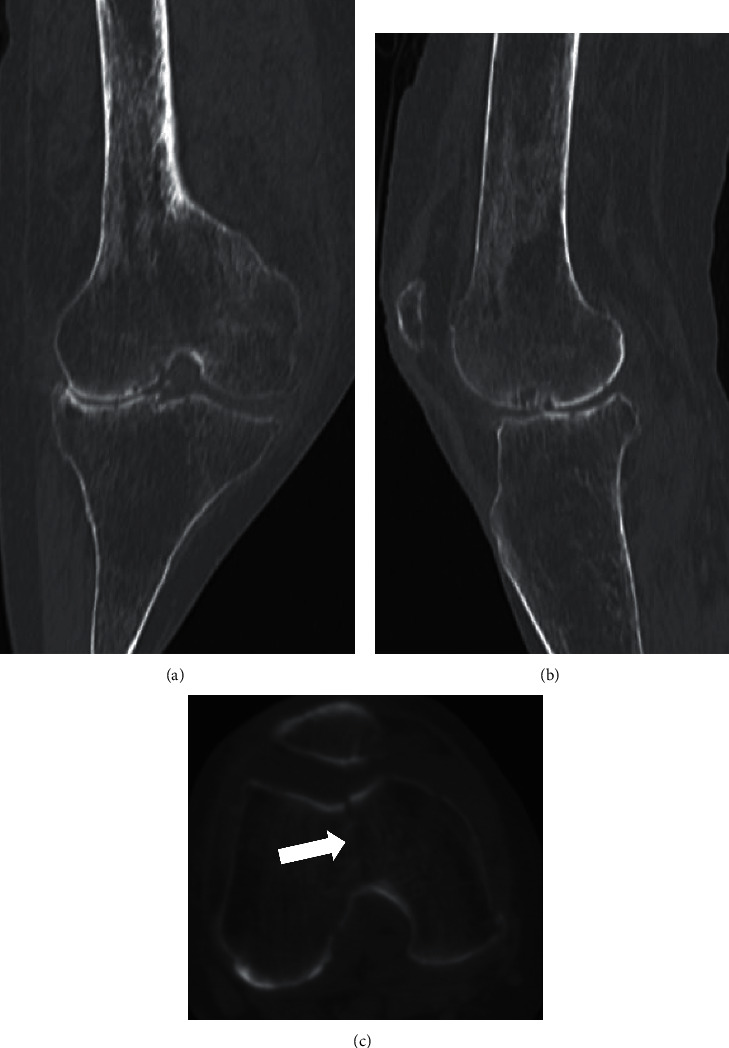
Two dimensional computed tomography (2DCT) scan demonstrating a fracture line from the trochlea to the femoral groove (AO Foundation/Orthopedic Trauma Association classification: type B2) (white and black arrow). (a) Coronal view, (b) sagittal view, and (c) axial view.
